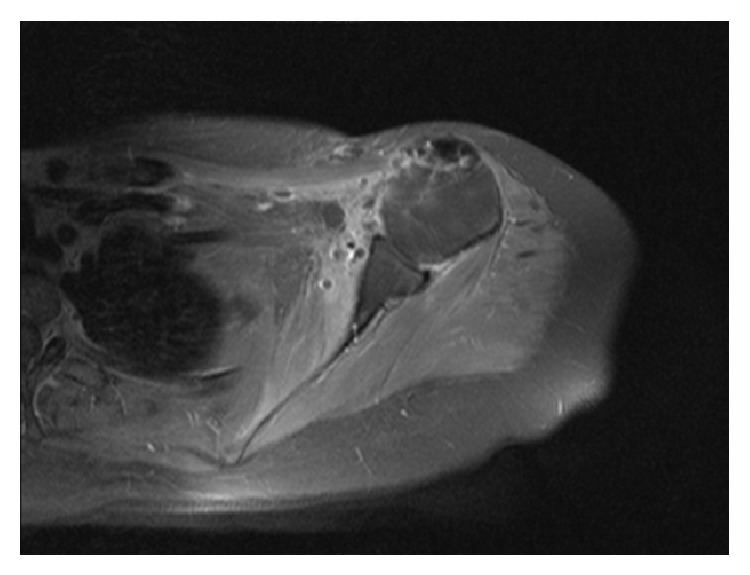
Postoperative MRI showing the anterior shoulder reconstruction with viable pedicle flap of pectoralis minor.
